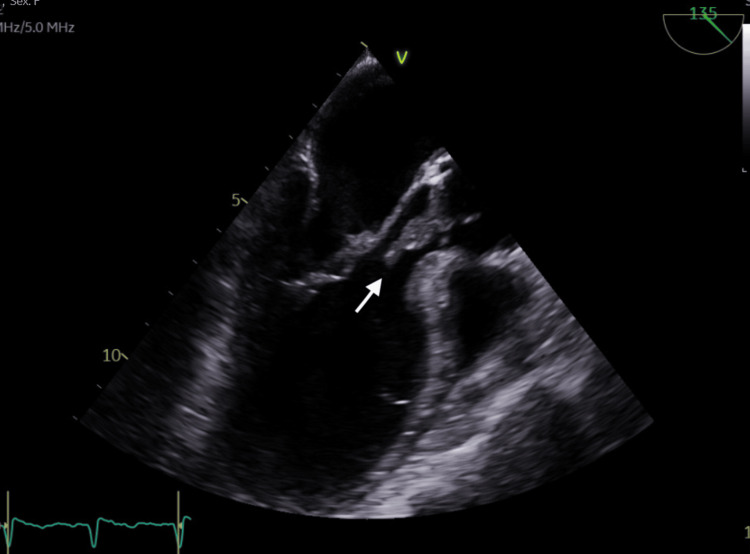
Transesophageal echocardiogram; mid-oesophageal long axis view in diastole showing a 0.63 cm x 1.59 cm vegetation (white arrow) attached to the non-coronary cusp.
